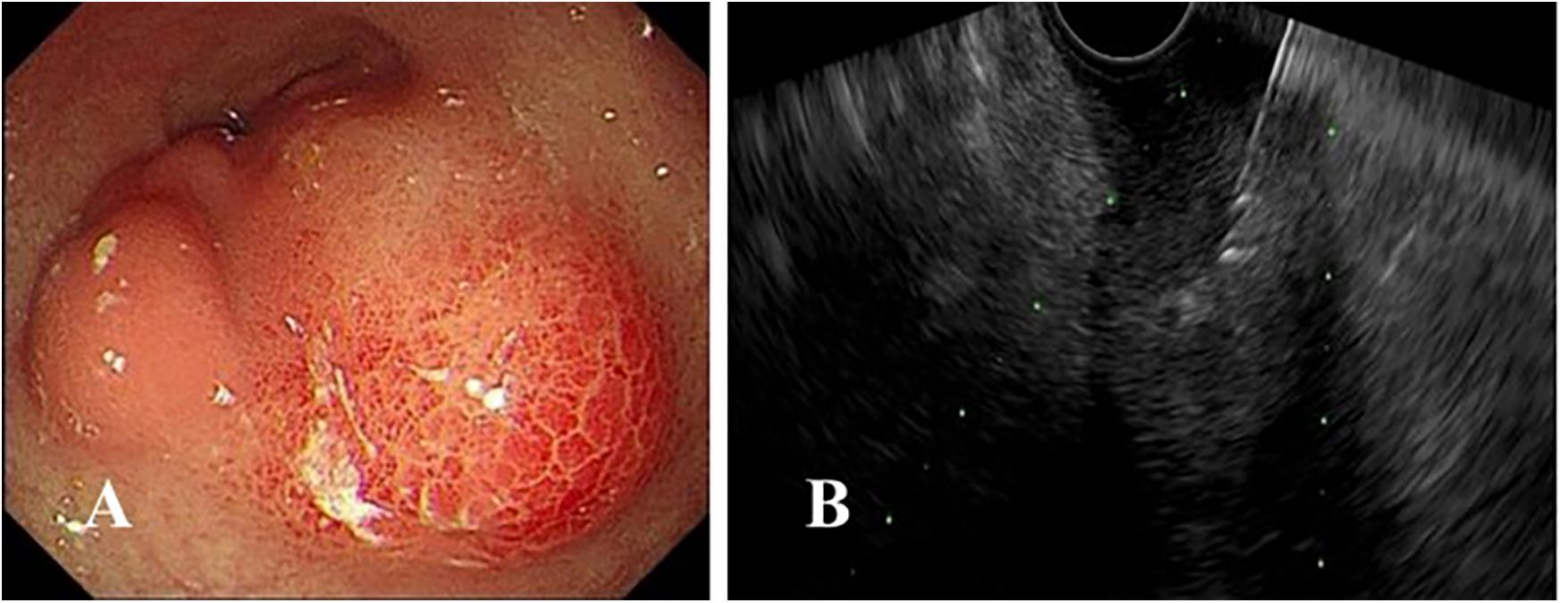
(A) Endoscopic findings revealing an irregular submucosal mass in the gastric antrum with overlying mucosa appearing congested and edematous; (B) endoscopic ultrasound-guided fine-needle aspiration was performed.
